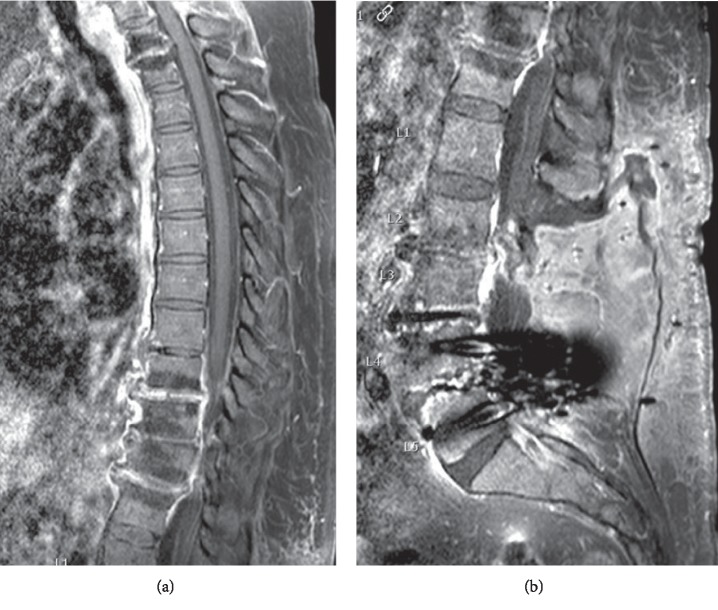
Thoracic (a) and lumbar (b) spine MR images demonstrate some enhancement within the C7–T1 acet joints as well as the T1–2 and T9–12 intervertebral discs. There is also ventral epidural enhancement from T9 to T12 and some enhancement within the lumbar surgical site.
